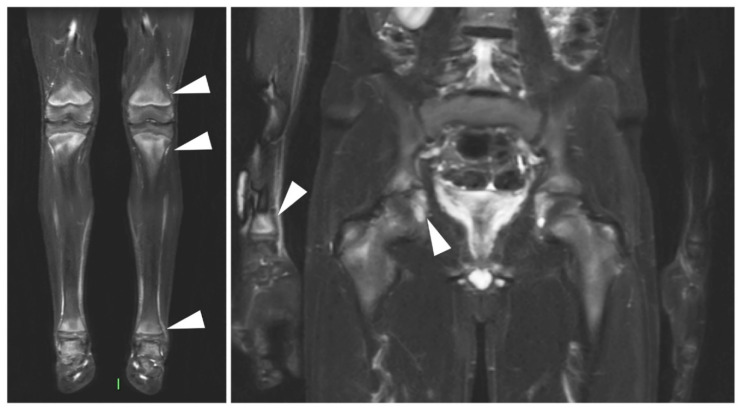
MRI in CRMO. Coronal T2 weighted with fat saturation images of a whole-body MRI in a 12 year old boy with chronic recurrent multifocal osteomyelitis (CRMO). There is evidence of bilateral bone marrow edema in the distal femur, distal/proximal tibia and talus, triradiate cartilage, and unilateral BME on the right distal radius (indicated by arrowheads. In the case of bilateral lesions, only one side was annotated).
